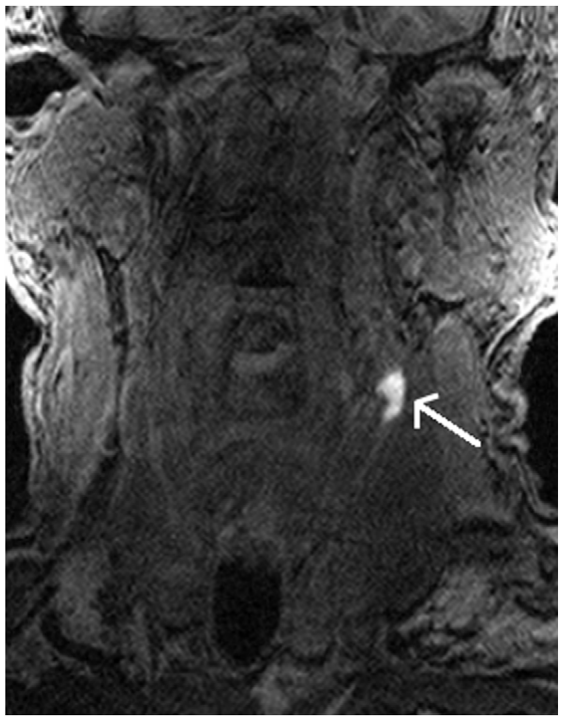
MRI scan showing the presence of carotid plaque hemorrhage seen in a coronal Section, (white arrow).
